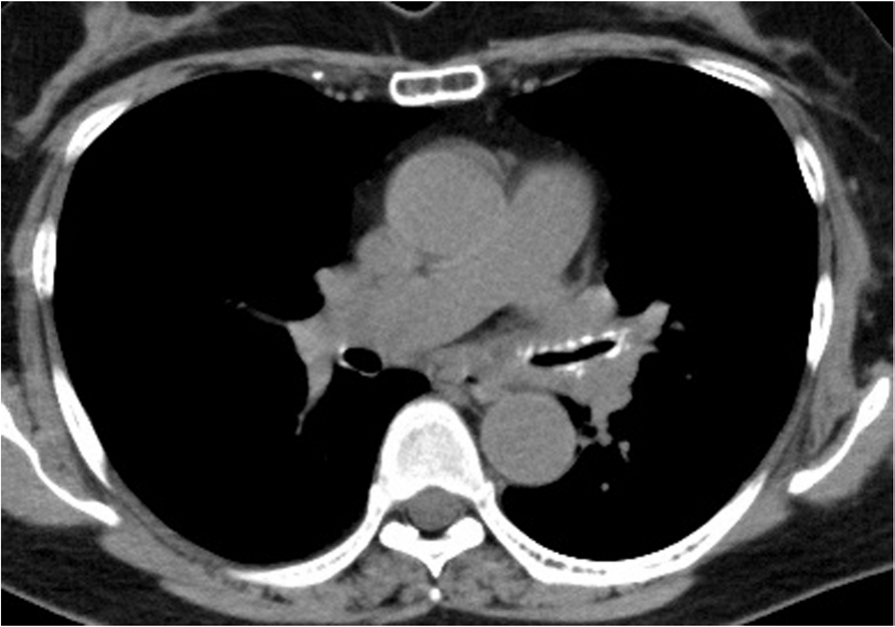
Chest CT imaging on admission showed a marked stenosis from the left main bronchus to the inferior lobe with suspected degeneration of cartilage
